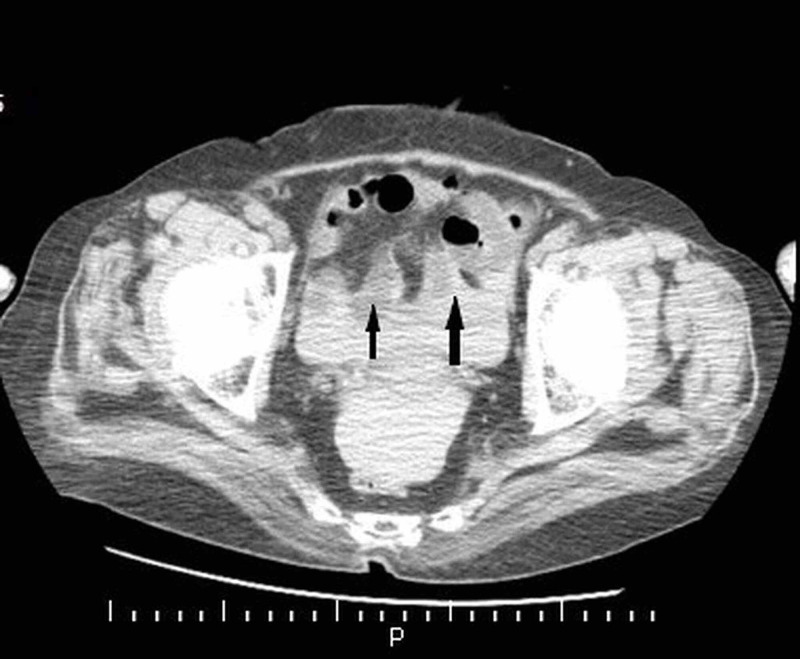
Abdomen computed tomography image: high-density fluid image around intra-abdominal organs compatible with intra-abdominal hemorrhage (black arrows)
